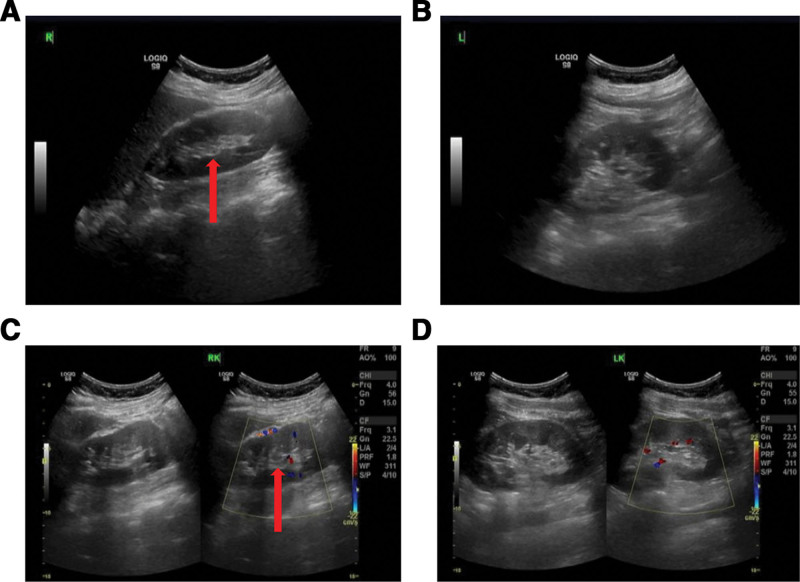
Serial ultrasonographic surveillance demonstrates absence of local recurrence in the first postoperative year after complete resection of a right-sided retroperitoneal tumor.
